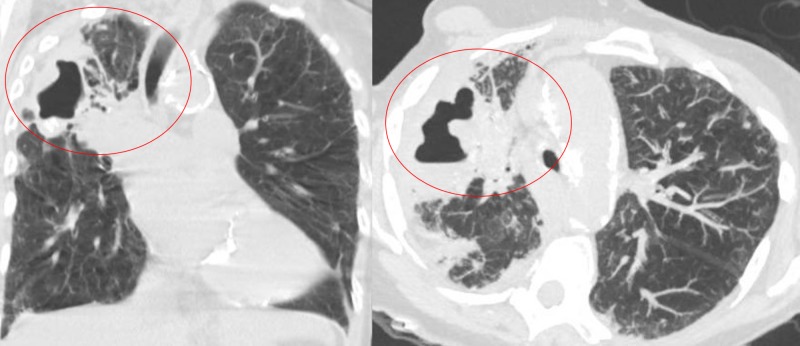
Repeat computed tomography of chest showing right upper lobe consolidation with slight interval enlargement of the right upper lobe cavitary lesion (circled)
